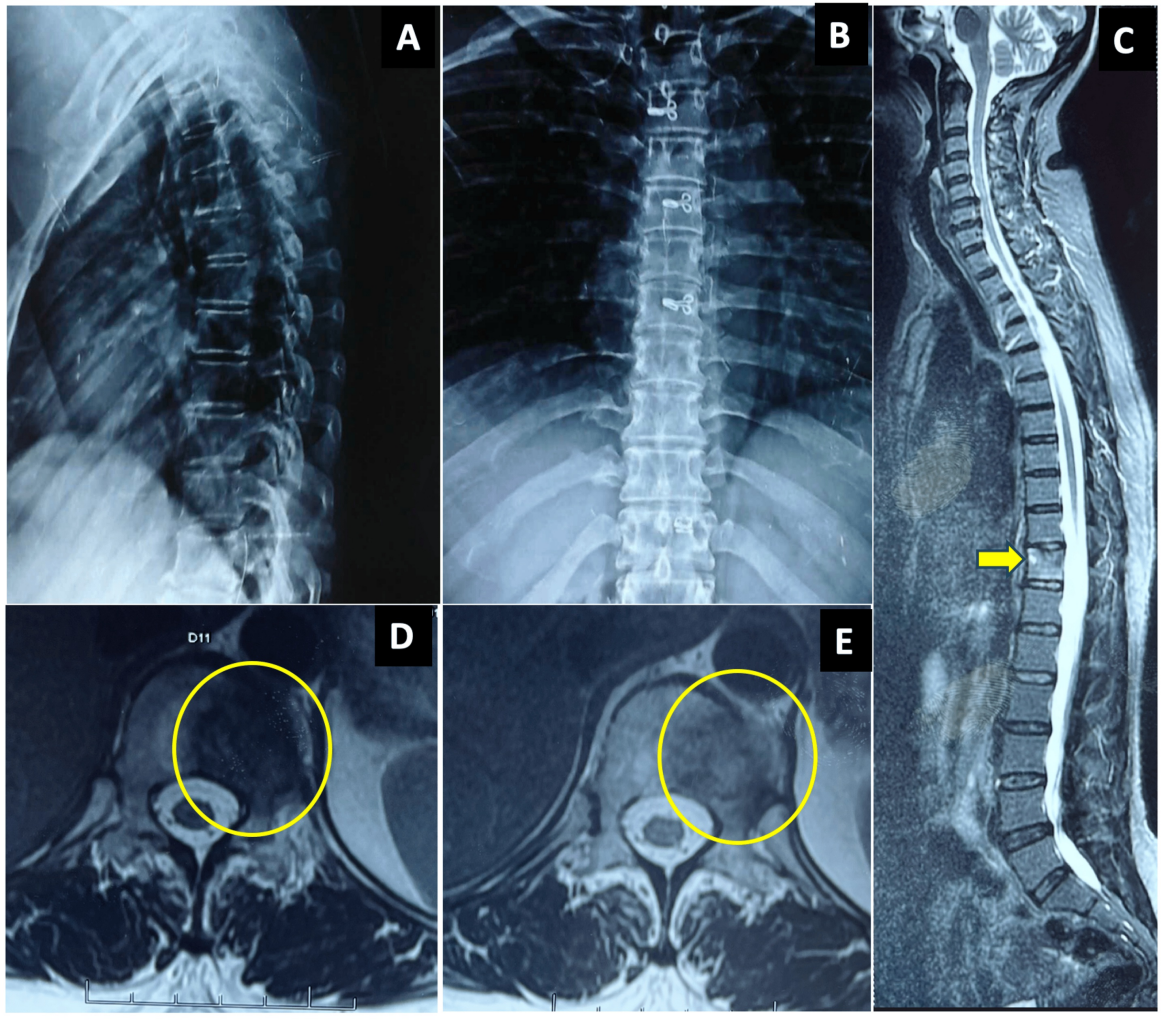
Preoperative radiographs of the patient. The patient’s antero-posterior radiograph (A) and lateral radiograph (B) show no significant findings. The MRI images show sagittal cuts (C) with the T11 tumor (yellow arrow) and MRI axial cuts (D, E) with the tumor inset (yellow circle). MRI: magnetic resonance imaging
